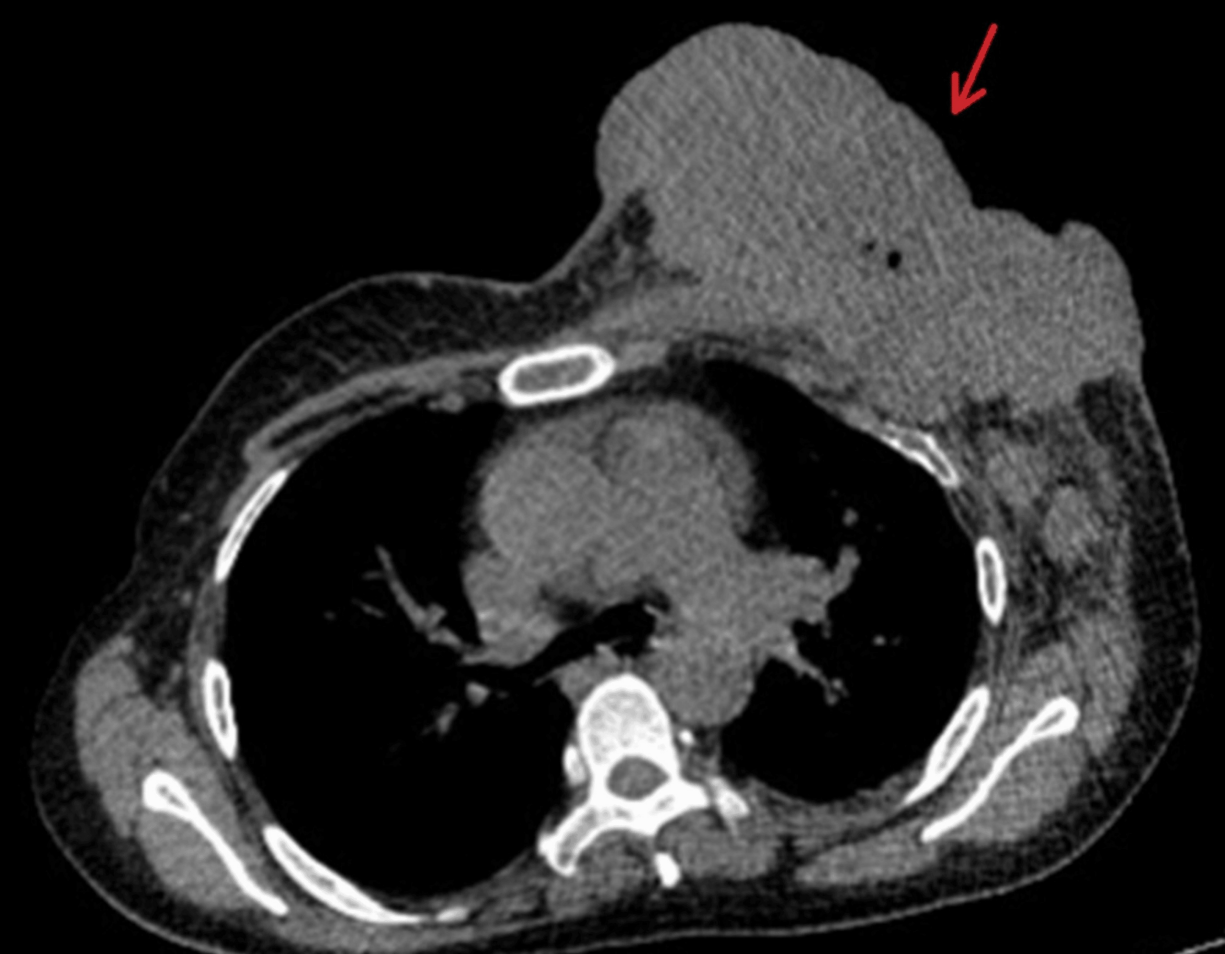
Axial contrast-enhanced CT of the chest showing advanced left breast malignancy A large, 16 cm multinodular mass was seen involving the left breast, with overlying skin ulceration and infiltration of the surrounding soft tissue. Multiple enlarged axillary lymph nodes were present (10-40 mm), consistent with locally advanced breast cancer.
